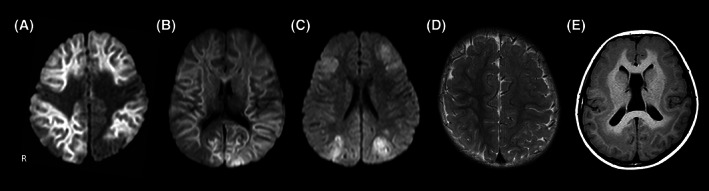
Brain MRI findings, A, AESD: Diffusion‐weighted imaging (DWI) image shows high signal intensity in the bilateral diffuse subcortical white matter, with relatively central sparing in the acute phase. B, AESD: DWI image shows bilateral diffuse subcortical high signal intensity without central sparing in the acute phase. C, HSES: DWI image shows symmetrical abnormally high signal intensity in the cortex and subcortical white matter in the acute phase. D, FCD: T2‐weighted image shows left hemispheric volume loss, abnormal cortical thickening, and blurring of the gray‐white matter junction. E, SBH: T1‐weighted image shows bilateral subcortical heterotopic gray matter in the posterior region fused with the pachygyric cortex in the anterior region. AESD, acute encephalopathy with biphasic seizures and late reduced diffusion; DWI, diffusion‐weighted imaging; FCD, focal cortical dysplasia; HSES, hemorrhagic shock and encephalopathy syndrome; MRI, magnetic resonance imaging; R, right; SBH, subcortical band heterotopia
